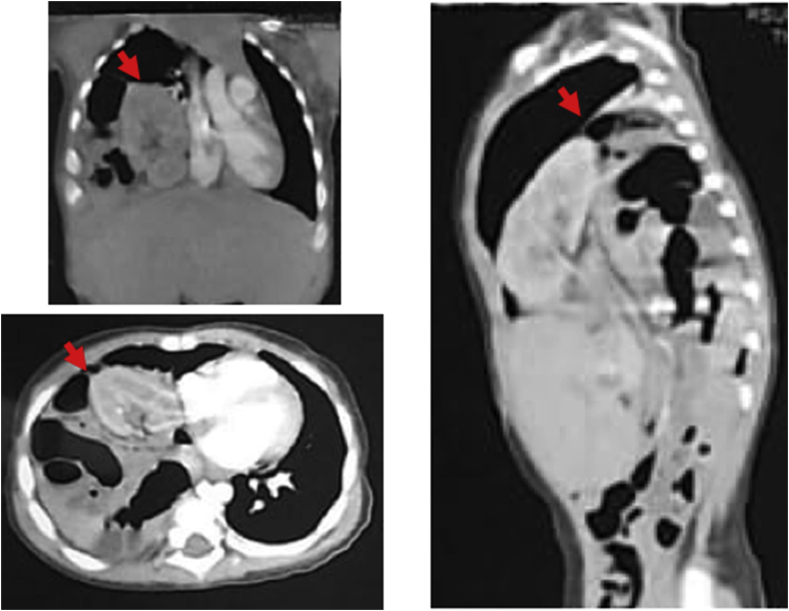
CT scanning exhibited the elevation of right diaphragmatic dome, with the 7th segment of the liver and transverse colon adhered to the right diaphragmatic dome.
